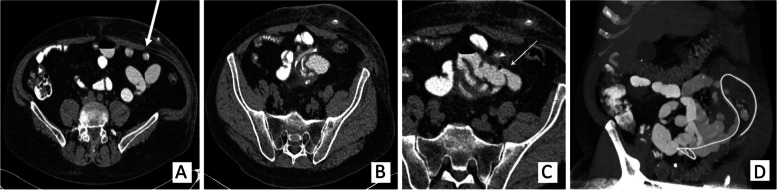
CT scan of the abdomen after oral opacification: axial views confirming the intraluminal trajectory of the catheter (thick arrow) (A). Fat infiltration around the distal extremity of the catheter (B) and pneumoperitoneum with extraluminal gas (thin arrow) (C). Coronal maximal intensity projection view showing the course of the catheter (D)
